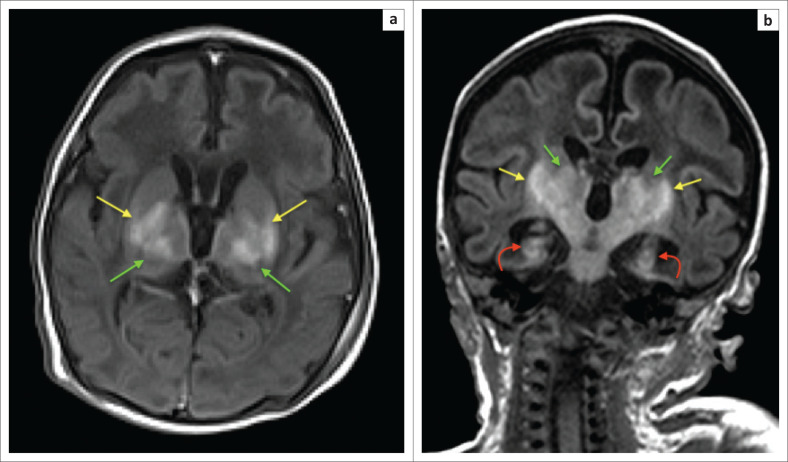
Two-week-old neonate born with grade 3 hypoxic ischemic brain injury and neonatal seizures. (a) An axial T1-weighted sequence showing signal shortening at the dorsal putamina (yellow arrows) and ventral thalami (green arrows). (b) Note, in addition to the basal ganglia and thalamic changes, the hyperintense signal and volume loss at both hippocampi (red arrows).
